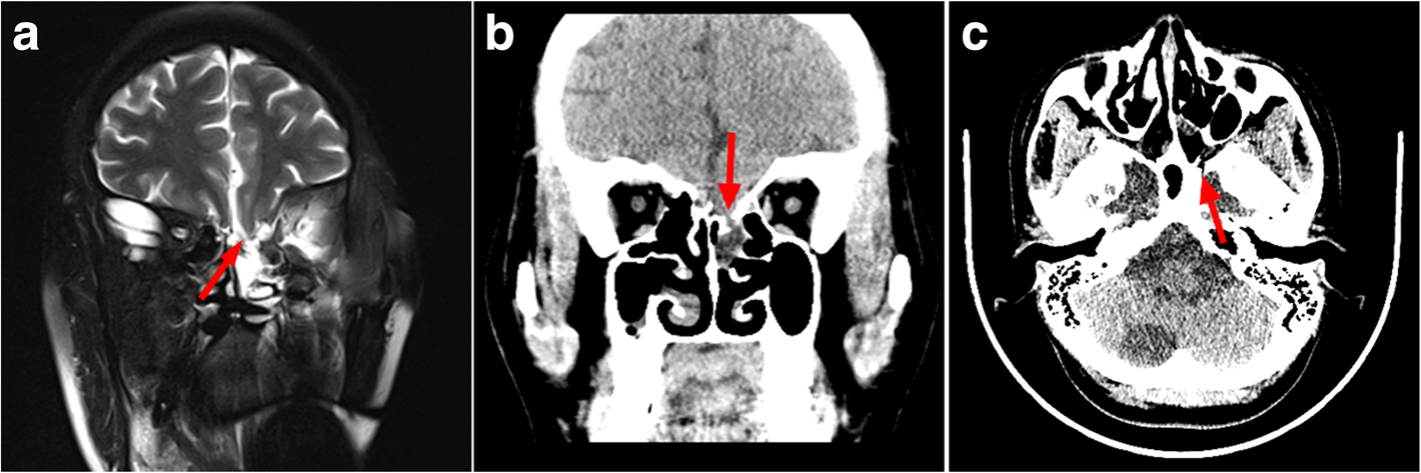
Images obtained 9 months after the operation. a T2-weighted coronal MR image. Arrow shows that the left anterior cranial fossa is communicated with the left ethmoid sinus. b and c The coronal and axial high-resolution bone window noncontrast CT images: Arrow indicates that there is a poor continuity of bone in the left ethmoid
